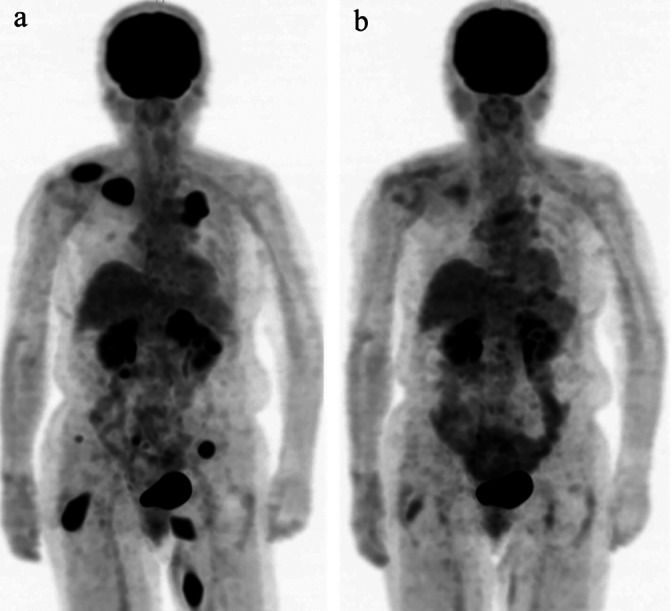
(a) Positron emission tomography‐computed tomography (PET‐CT) scan before the initiation of alectinib treatment showing a primary lesion in the left upper lung and multiple lung metastases. (b) PET‐CT scan obtained two months after the initiation of alectinib shows tumor shrinkage in the primary lesion and reduction in multiple metastatic sites
